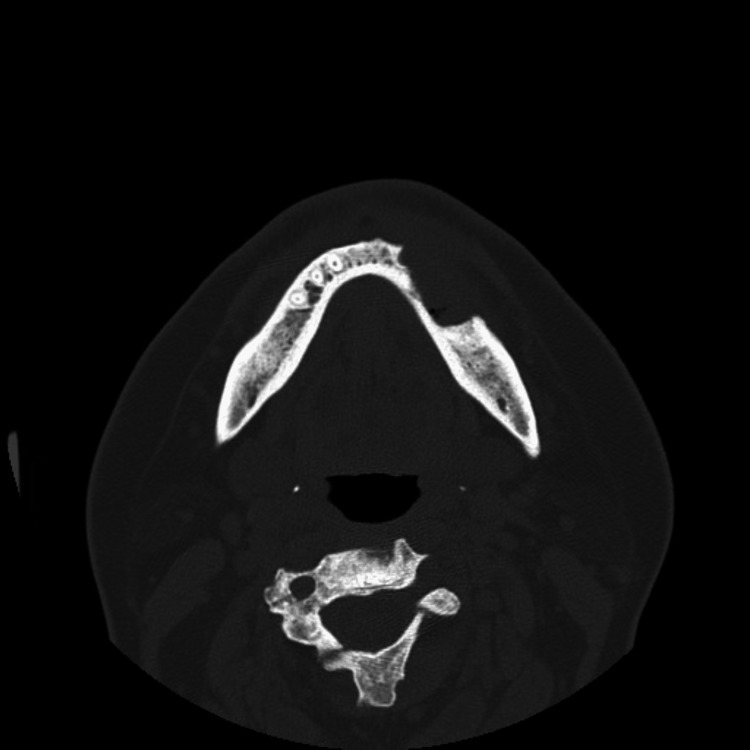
Post-Operative Axial CT Scan for the Surgical Site
